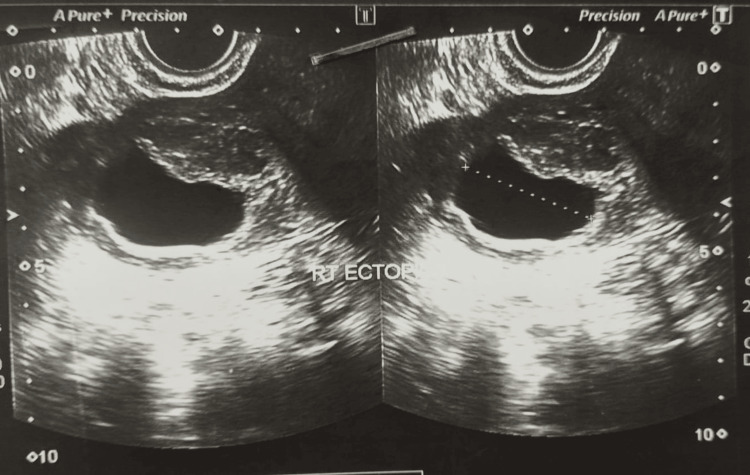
Ultrasound image demonstrating a right-sided ectopic pregnancy
